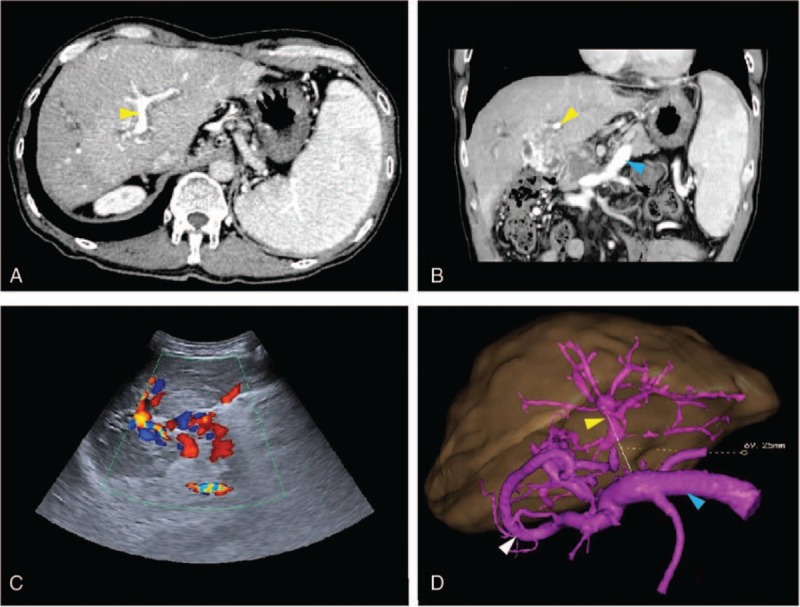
Preoperative enhanced CT scan (A, B) and Doppler US images (C) showed a patent LPV (yellow arrow), SV (blue arrow) and the confluence of the SMV and SV (D). On 3D reconstruction, an obvious varix presented (white arrow). The distance between the LPV and SV, measured to facilitate surgery, was 69.25 mm (yellow line) (D). CT  =  computed tomography, LPV  =  left portal vein, SMV  =  superior mesenteric vein, SV  =  splenic vein, 3D  =  three dimensional, US  =  ultrasound.
